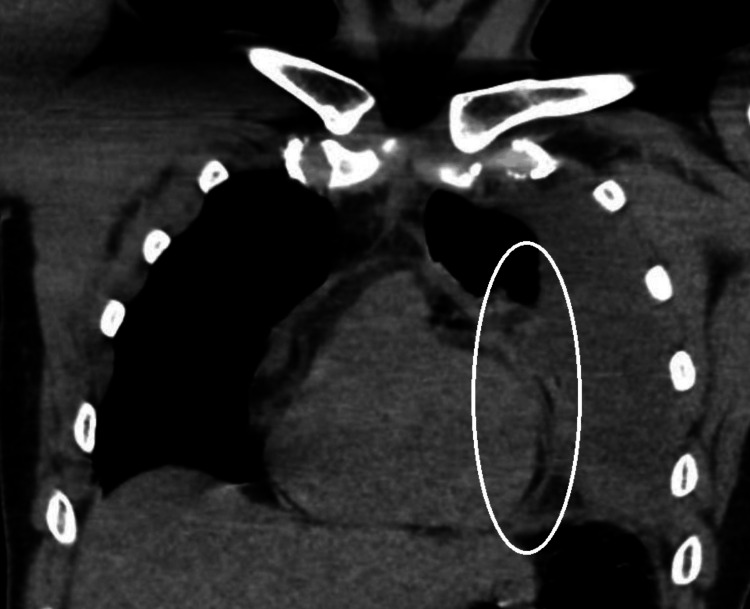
Coronal view of chest computed tomography (CT) on day 1 demonstrating a large loculated left pleural effusion with direct anatomical proximity to the pericardium. This close spatial relationship between the pleural and pericardial compartments may have clinical implications, particularly in the context of intrapleural fibrinolytic therapy. At this time, no pericardial effusion was observed.
